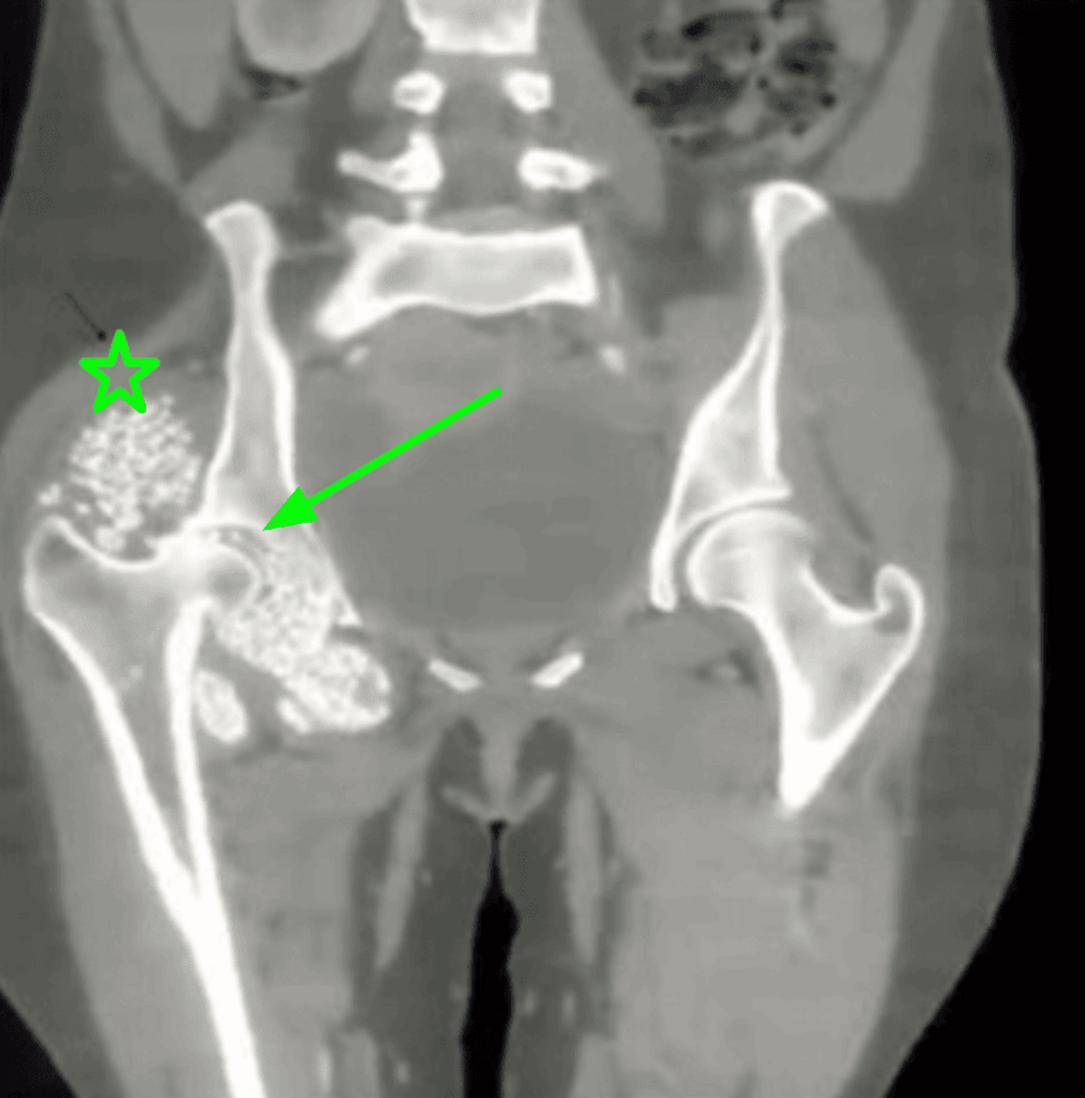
CT of the pelvis, including the bilateral proximal lower limb bone window Arrow: Signs of development of secondary osteoarthritis were also evident by articular sclerosis of the acetabulum with subarticular cystic areas, irregularities, and small erosions; Star: Gluteus medius in comparison to the left side showed a reduced bulk likely secondary to atrophy
